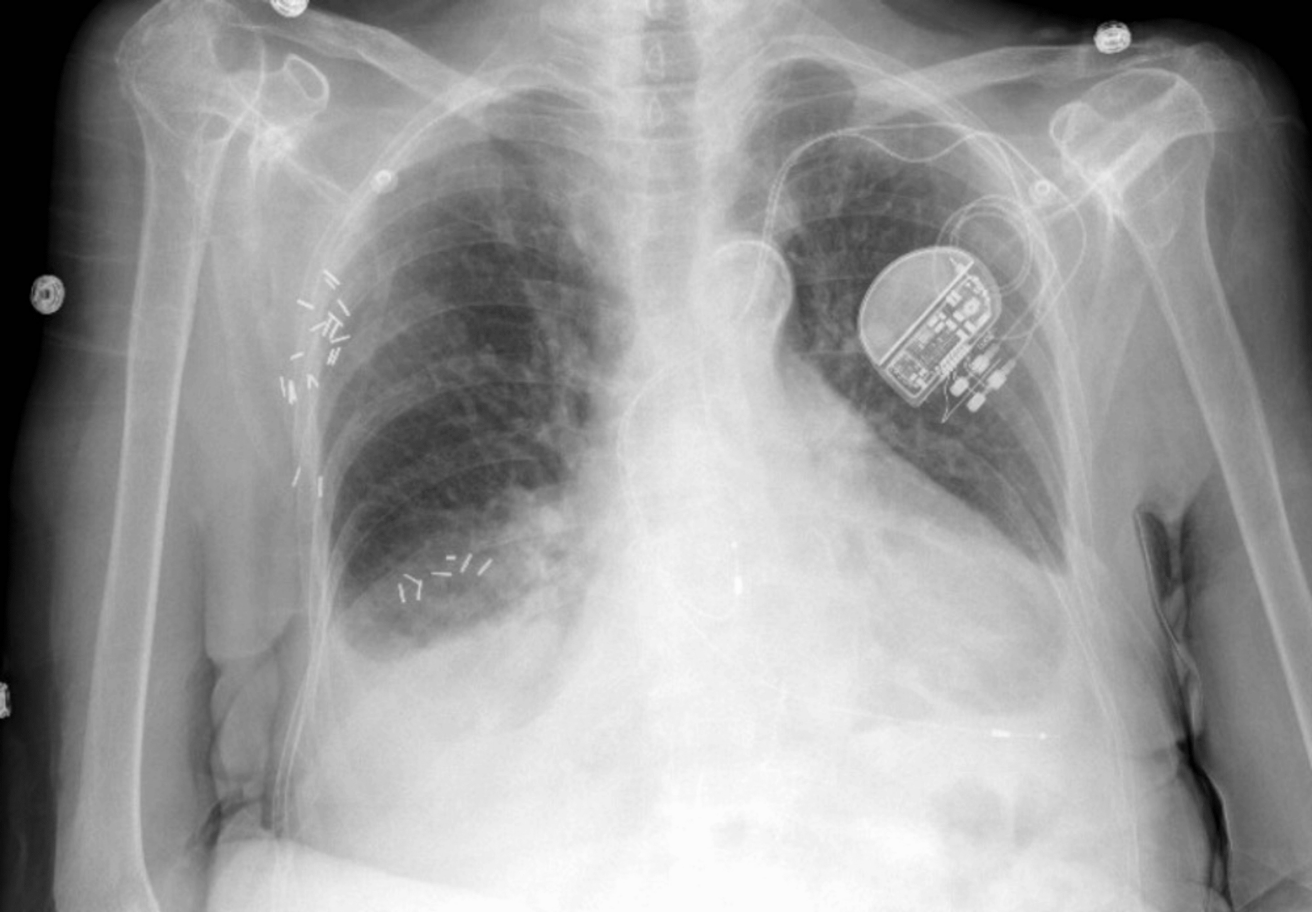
Chest X-ray showing mild pulmonary vascular congestion with opacity at bilateral lung bases.
